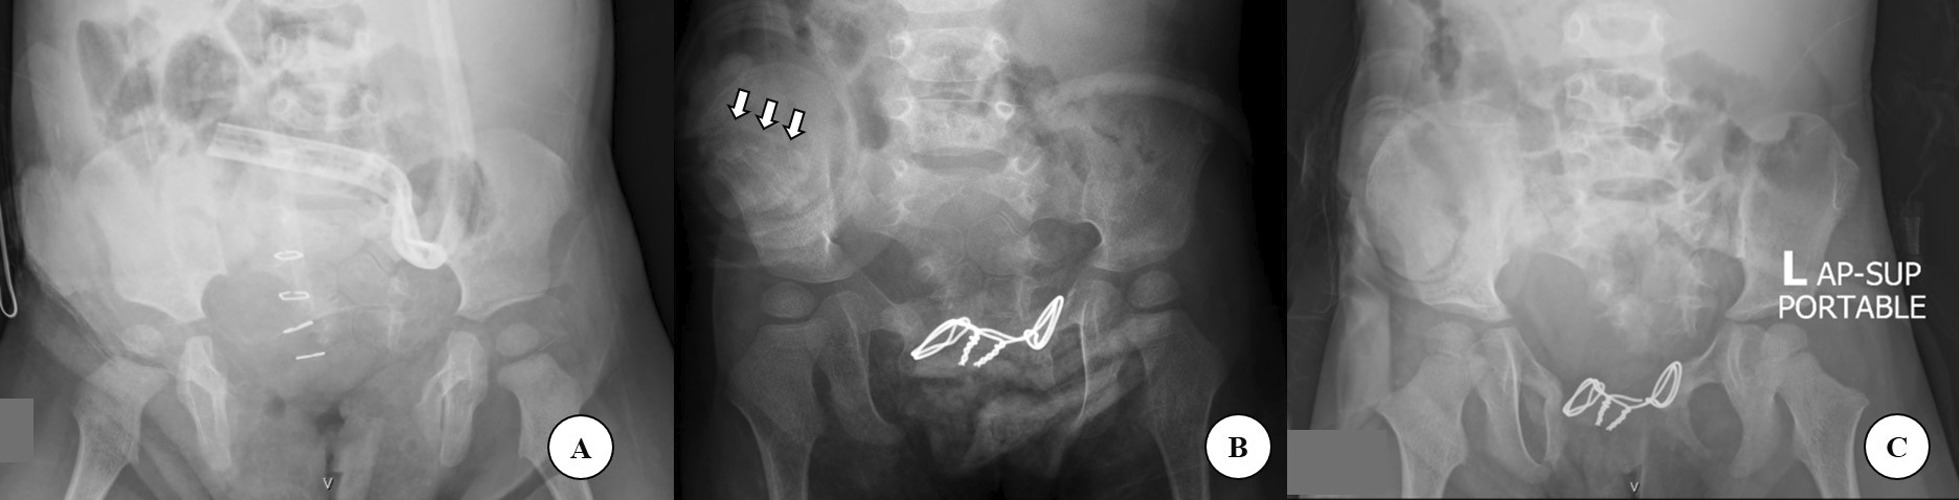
A–C Radiographs of a 16-month-old girl at preoperative (A), postoperative (B), and at 3 years follow-up, white arrows emphasized osteotomy sites (C), after underwent bilateral bayonet osteotomy and pubic symphysis approximation with wiring
